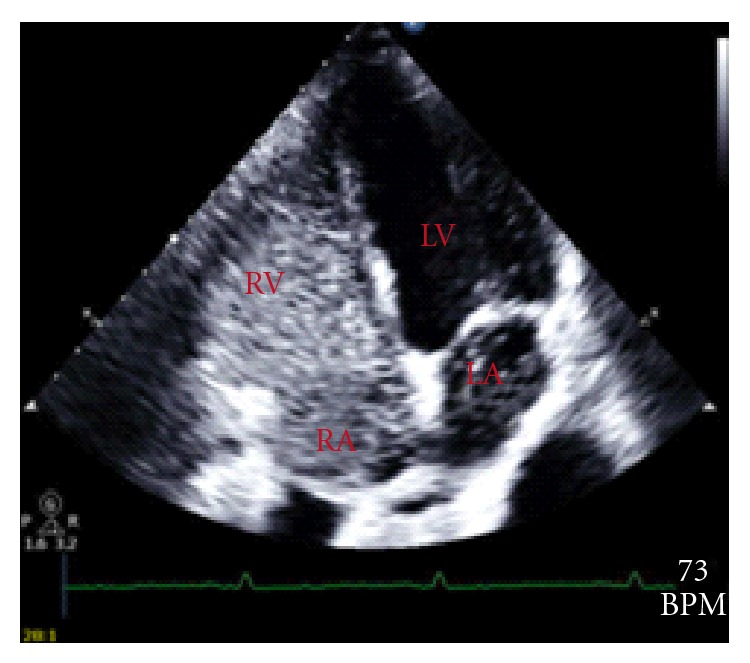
Contrast-enhanced echocardiography showing the appearance of microbubbles in the left atrium 6 cycles after they first appeared in the right.
